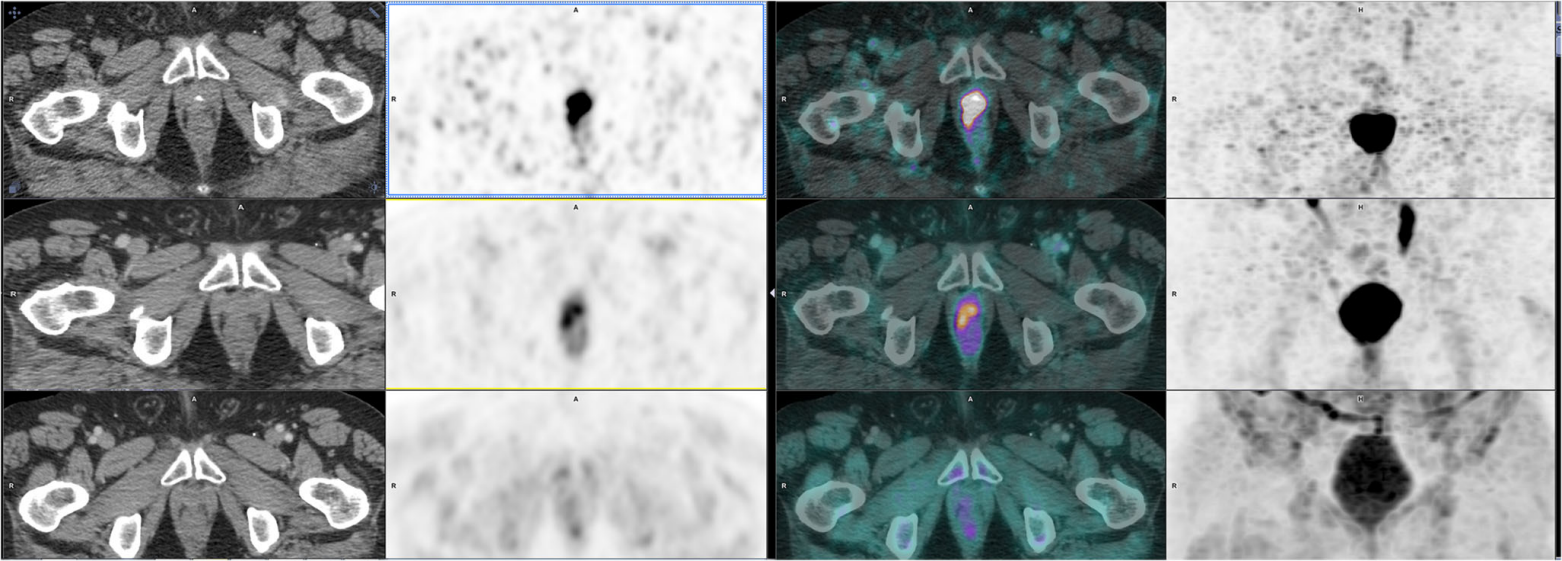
This 64-year-old patient underwent FCH PET/CT for BCR (sPSA = 0.67 ng/mL), 3 years after total prostatectomy (pT3 N0 M0). a FCH PET/CT (lower row of images; from left to right: CT, PET, fused PET/CT fusion, and MIP) showed a mild doubtful focus (SUVmax = 3.55) in the urethral anastomosis on the right side. b
